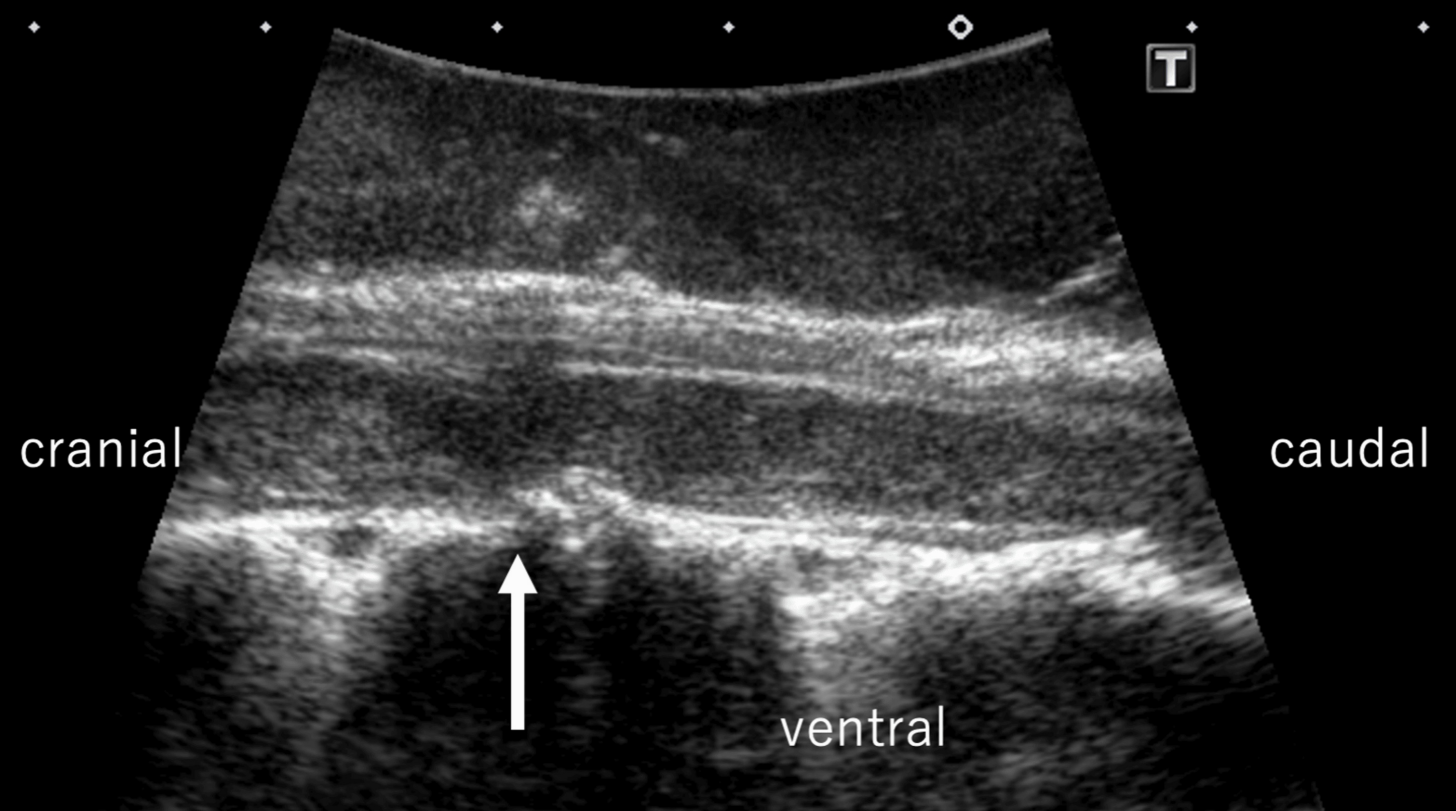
Ultrasound image taken during surgery Intraoperative ultrasound image. The spinal cord is shifted anteriorly, with expansion of the posterior subarachnoid space and disappearance of the anterior subarachnoid space (arrow).
